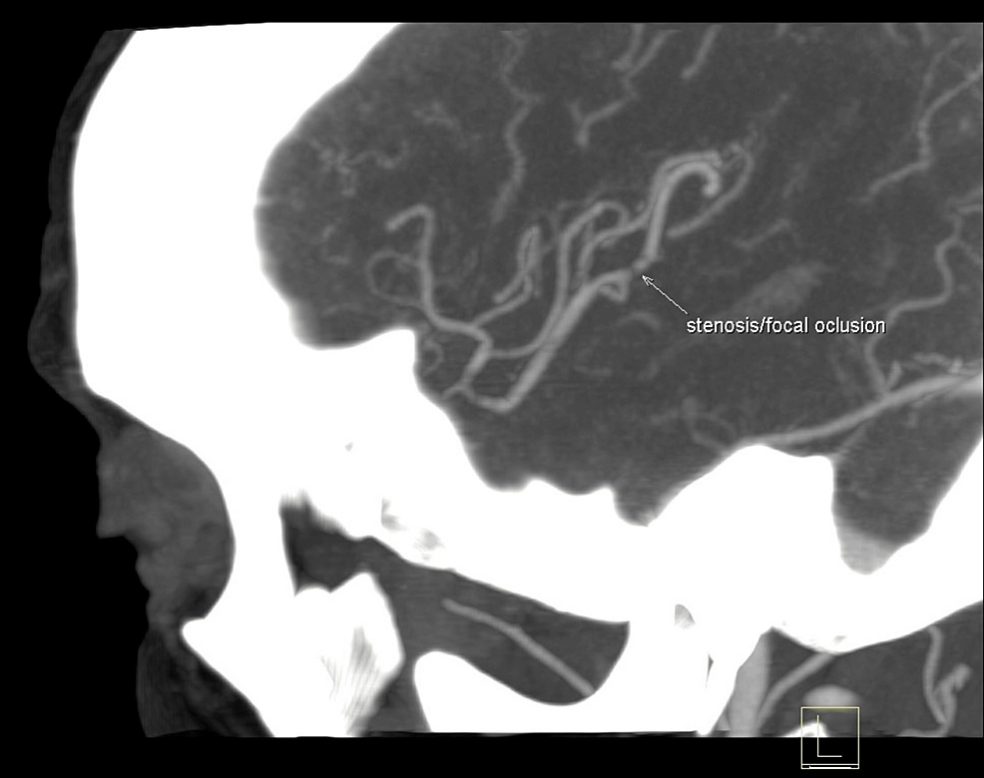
Head computed tomography with angiogram showing a focal occlusion at M2-M3 of the left medial cerebral artery
